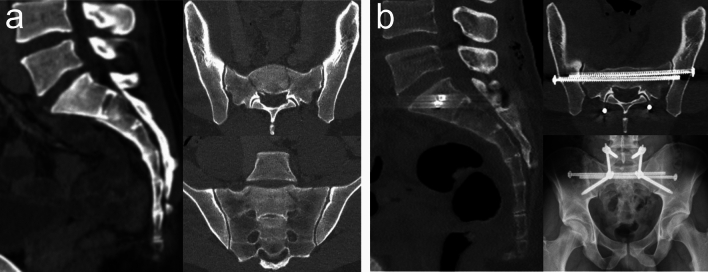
Spinopelvic dissociation injury (SPDI; Type 2 TLSI). A 29-year-old male paraglider sustained a SPDI with sacral nerve root dysfunction (hypoesthesia and impaired sharp-dull discrimination in the S2-S5 dermatomes but preserved voluntary anal contraction) and a right-sided open lower leg shaft fracture following a fall from height. a Preoperative CT images showing a U-shaped Type 2a SPDI with the transverse fracture component at S2. b Postoperative CT images and an AP radiograph after closed reduction in the prone position and percutaneous TLPF demonstrate anatomic reduction
